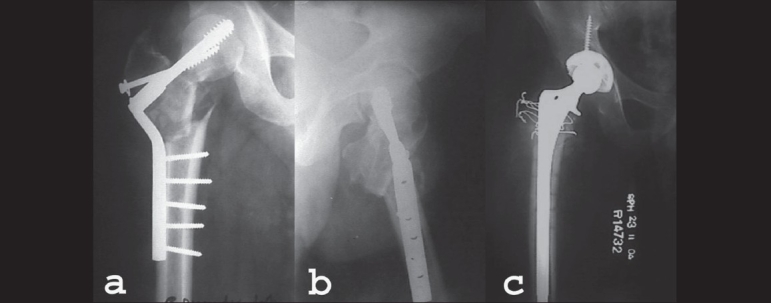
(a) AP radiographs of 38yrs old gentleman. Fracture neck of femur Rt.side treated with primary Powell's osteotomy and fixation with double angle barrel plate. (b) Lateral radiograph of same patient showing implants cutting out and fracture fragments in malaligned position. (c) AP radiograph showing uncemented total hip arthroplasty done 14 months after the primary fixation surgery
